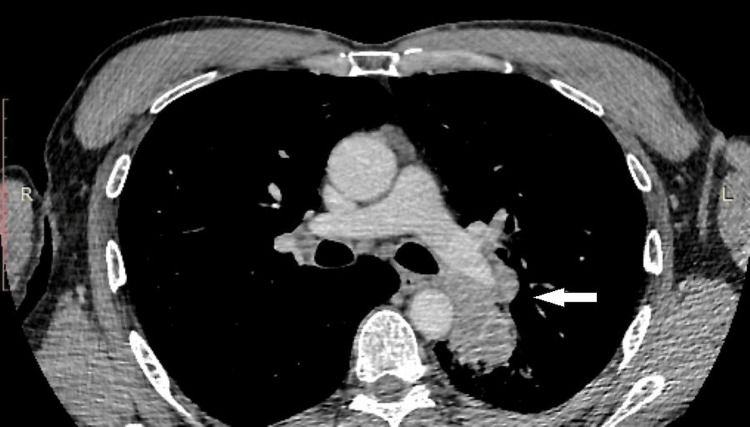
The axial section of the thoracic preoperative CT scan showing tumor relationship to the main pulmonary artery, descending aorta, and left main bronchus. CT: computed tomography
